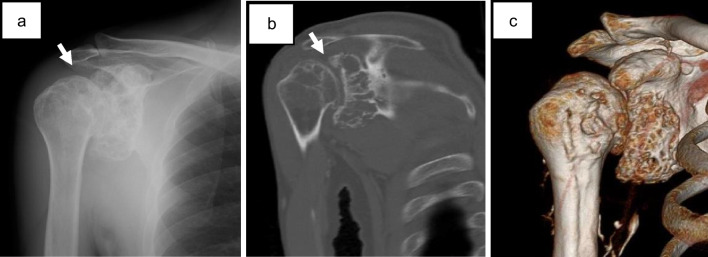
Radiography and CT images taken in our hospital. a Radiography reveals irregular osteolytic lesions with sclerotic changes in both the scapular and proximal humerus (arrow). b, c CT images show intramedullary osteolytic lesions with focal cortical destruction (arrow)
